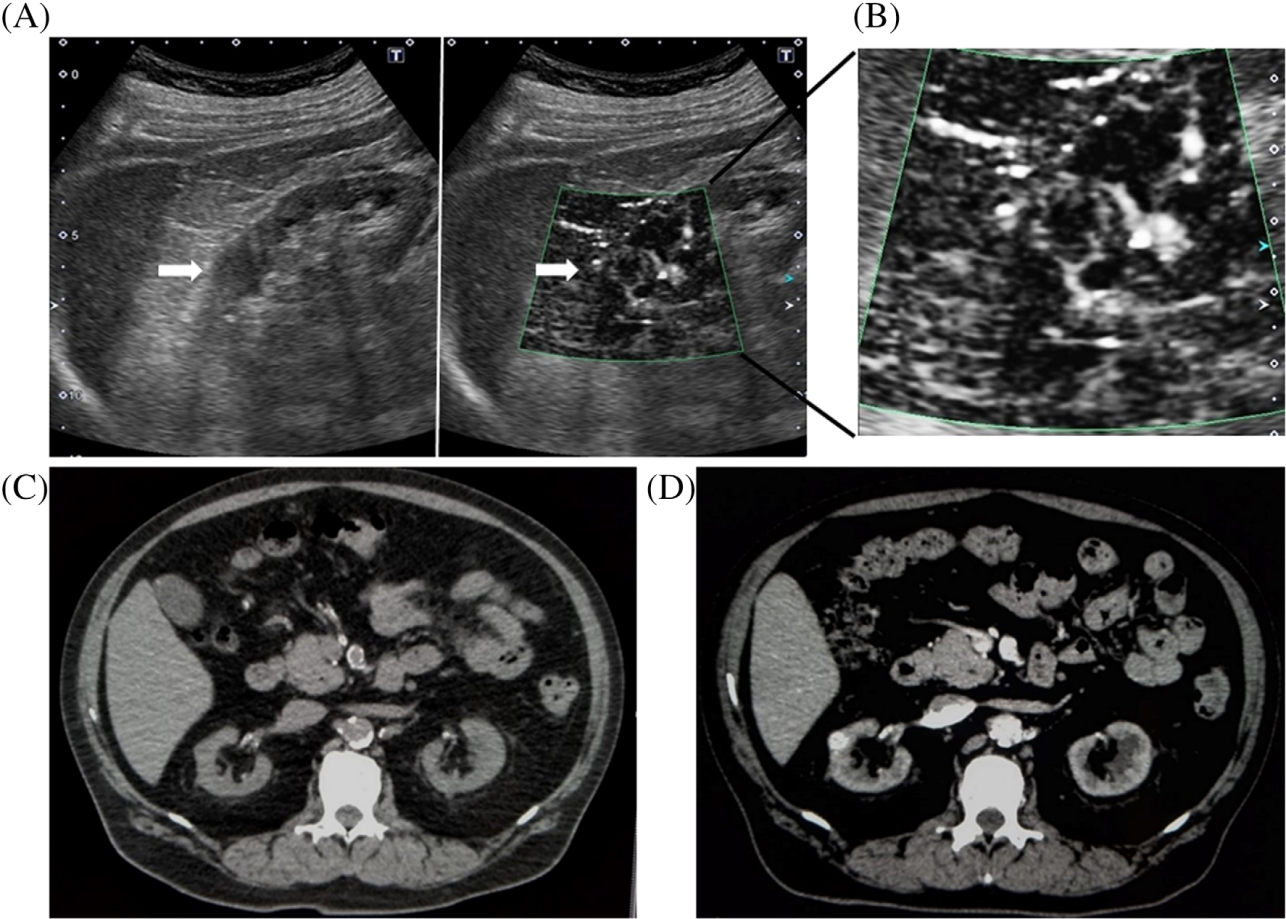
(a) B mode image in the ultrasonography. Oval mass is observed at the center part of the right kidney (17 mm × 16 mm in diameter) and it shows relatively low and homogeneous echo in the internal part (white arrow). (b) Monochrome superb microvascular imaging (mSMI) in the ultrasonography and its magnified image. Blood flow is sharply captured by mSMI of ultrasonography at margin and inside of the mass (white arrow), which was not detected at all with conventional color or power Doppler. (c) Nonenhanced CT image. The mass shows constant density compared with other part of the kidney and thereby it is not clearly detected. The protrusion of the mass is not clearly observed due to its small size (white arrow). (d) Dynamic contrast‐enhanced CT image. A nodular lesion is clearly detected at the center of the right kidney (white arrow) [Color figure can be viewed at wileyonlinelibrary.com]
